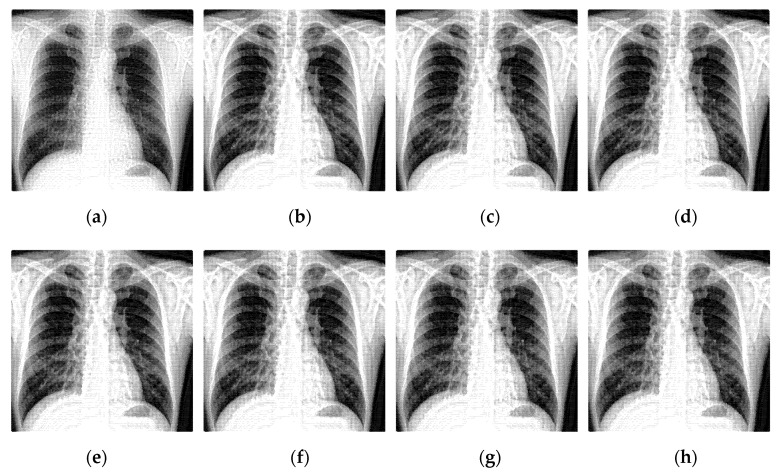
The enhanced effects of different shearing directions on X-ray image 1. In (a), the shearing parameters of each level are set to (4,4,4) and the shearing directions are (16,16,16). For (b–h), the decomposition scale was set to 4 levels, the shearing parameters of each level are set from (2,2,2,2) to (4,4,4,4), and the shearing directions are from (4,4,4,4) to (16,16,16,16). The number of shearing directions of each level was gradually increased.
